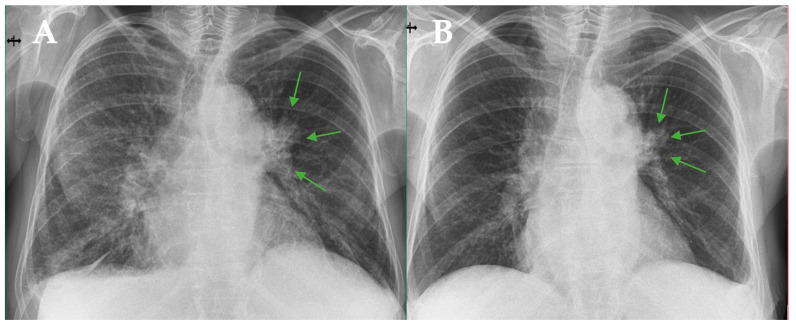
Posteroanterior chest radiographs. (A) At the initial evaluation. (B) Ten months after treatment initiation, showing a slight reduction in the size of the left hilar lesion, indicated by green arows. Immunosuppression is a major risk factor for various infections, including nontuberculous mycobacterial (NTM) infection. Patients with myelofibrosis treated with ruxolitinib are particularly vulnerable to mycobacterial infections due to impaired cellular immunity [1,2]. Ruxolitinib, a JAK1/2 inhibitor, has been associated with opportunistic infections, including tuberculosis and nontuberculous mycobacterial disease [2]. The increased susceptibility is thought to be related to modulation of T cell-mediated immune responses, particularly reduced regulatory and effector T cell function [3]. The distinctive feature of this case is the pseudotumoral radiologic appearance of *Mycobacterium avium* infection. Although MAC pulmonary disease typically presents with nodular bronchiectatic or cavitary patterns, mass-like consolidation has rarely been described and may mimic lung malignancy [4]. In immunocompromised hosts, altered granulomatous response and reduced necrosis may contribute to this atypical imaging pattern. A similar association between ruxolitinib therapy and atypical pulmonary infections has been previously reported [5]. This case highlights the importance of including NTM infection in the differential diagnosis of tumor-like pulmonary lesions in patients receiving JAK inhibitors, in order to avoid misdiagnosis and inappropriate oncologic management.
